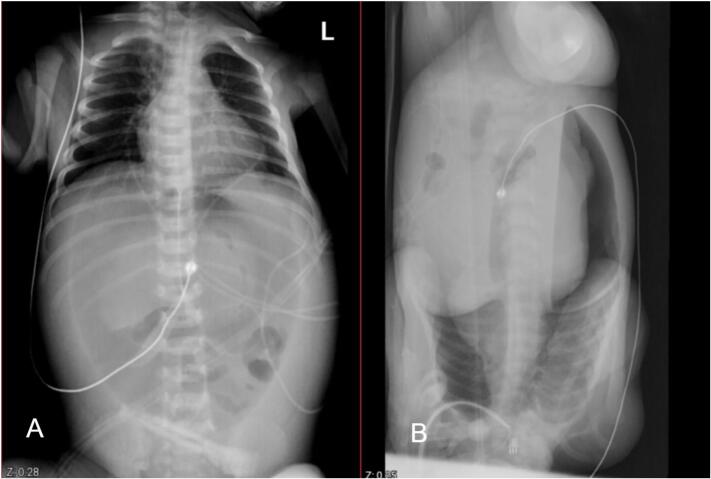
A: Plain abdominal X-ray showing football sign. B: Lateral decubitus abdominal X-ray demonstrating pneumoperitoneum.
